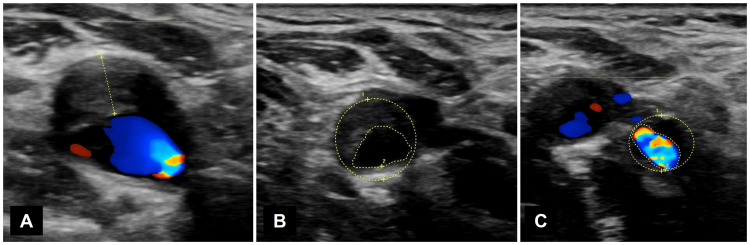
Carotid ultrasonography six months after the first visit showing atheromatous stenosis in the right internal carotid artery (A,B) and external carotid artery (C)
